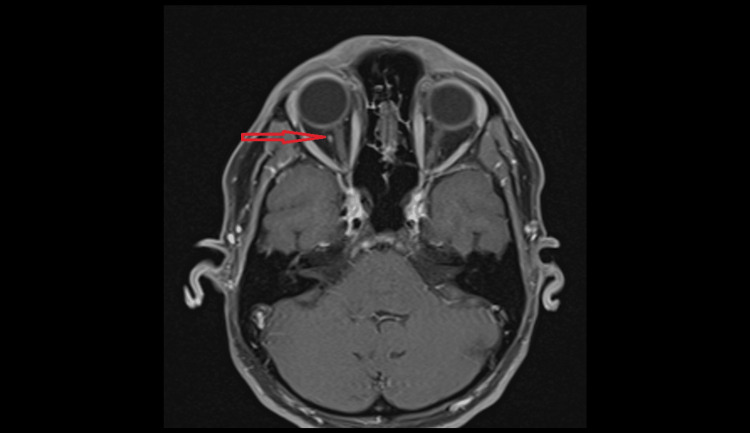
Axial view of a T1-weighted MRI post-contrast highlighting the same structure (red arrow) seen in Figure 4
